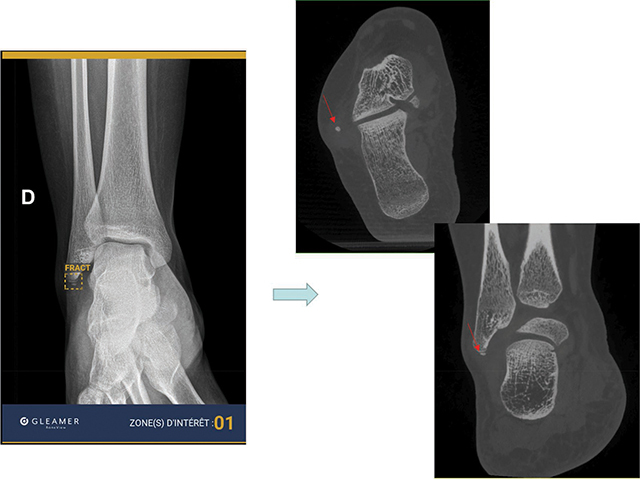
AI false positive response for fracture of the external malleolus, which CBCT confirmed to be a calcification.
